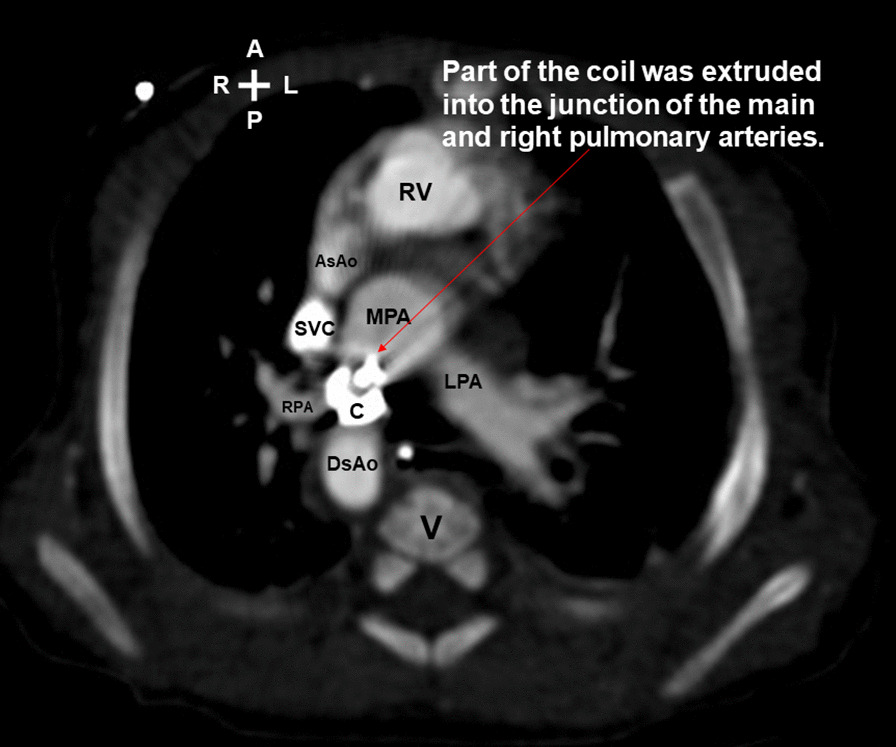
Computed tomography angiography (axial view) more clearly demonstrated that part of the coil was extruded into the junction of the main and right pulmonary arteries. *A* anterior; *AsAo* ascending aorta; *C* coil; *DsAo* descending aorta; *L* left; *LPA* left pulmonary artery; *MPA* main pulmonary artery; *P* posterior; *R* right; *RPA* right pulmonary artery; *RV* right ventricle; *SVC* superior vena cava; *V* vertebra
